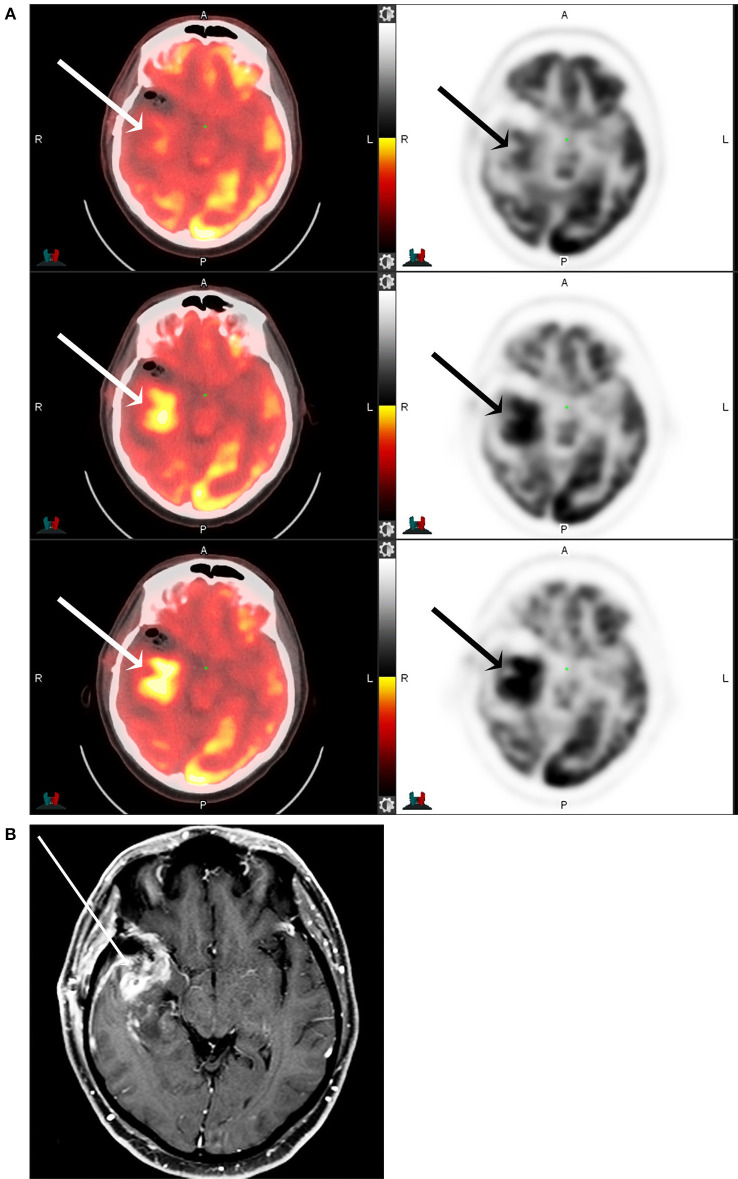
F18 FDG PET CT (A) and T1 post-contrast MRI of the brain (B) of a 53-year-old male with partially resected IDH-mutant, glioblastoma of the right anterior temporal lobe (arrows) at 1-, 5-, and 8-h following F18-FDG injection. The maximum tumor SUV, as well as the tumor to background ratios, are seen to increase with increasing time from the injection.
